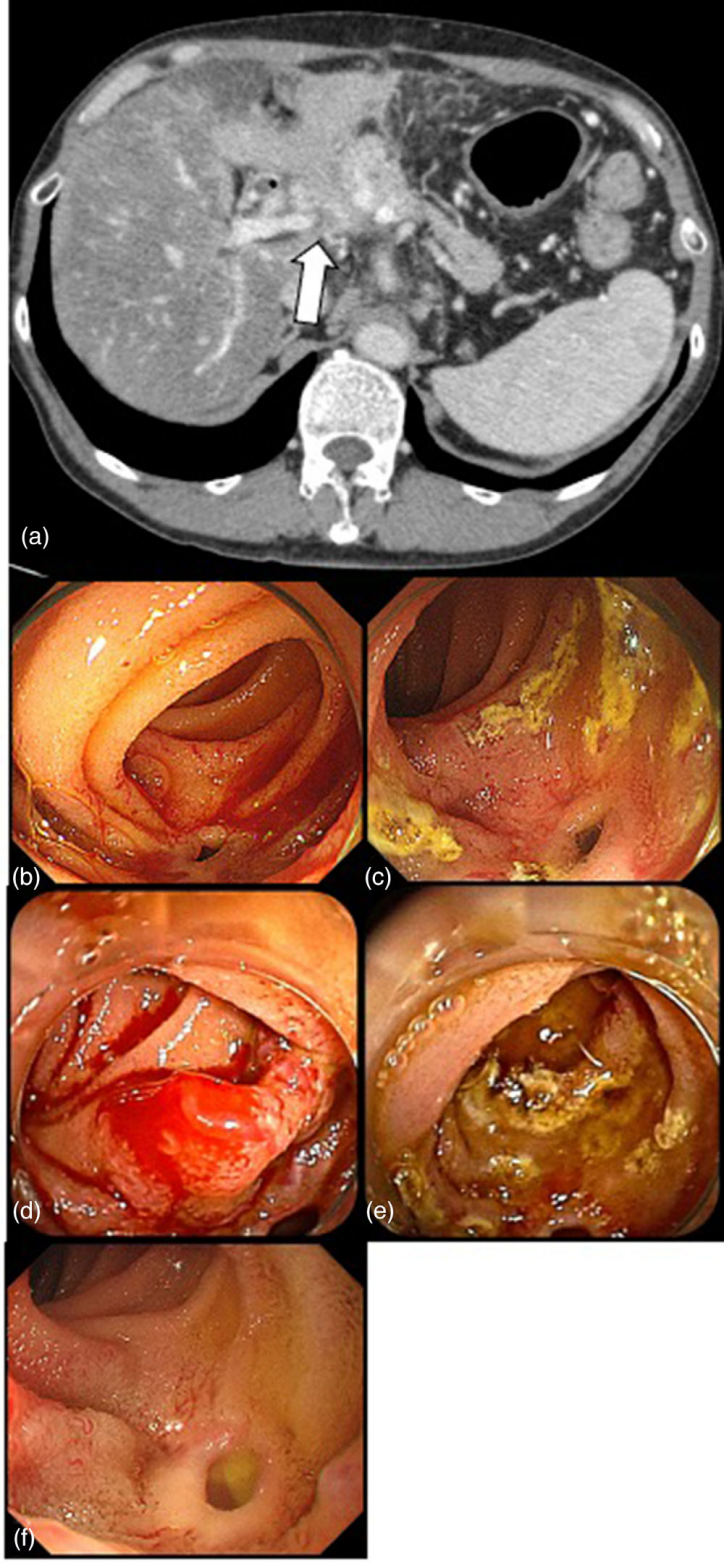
In case 1, contrast‐enhanced computed tomography showed portal vein stenosis (a). Endoscopic image shows the angiodysplasia‐like lesions around the hepaticojejunostomy and bleeding (b). We employed argon plasma coagulation (c). Three years after hemostasis, bleeding from the angiodysplasia‐like lesion around the hepaticojejunal anastomosis recurred (d). We employed argon plasma coagulation (e). Two years later, there was no further bleeding (f). White arrow: portal vein stenosis
